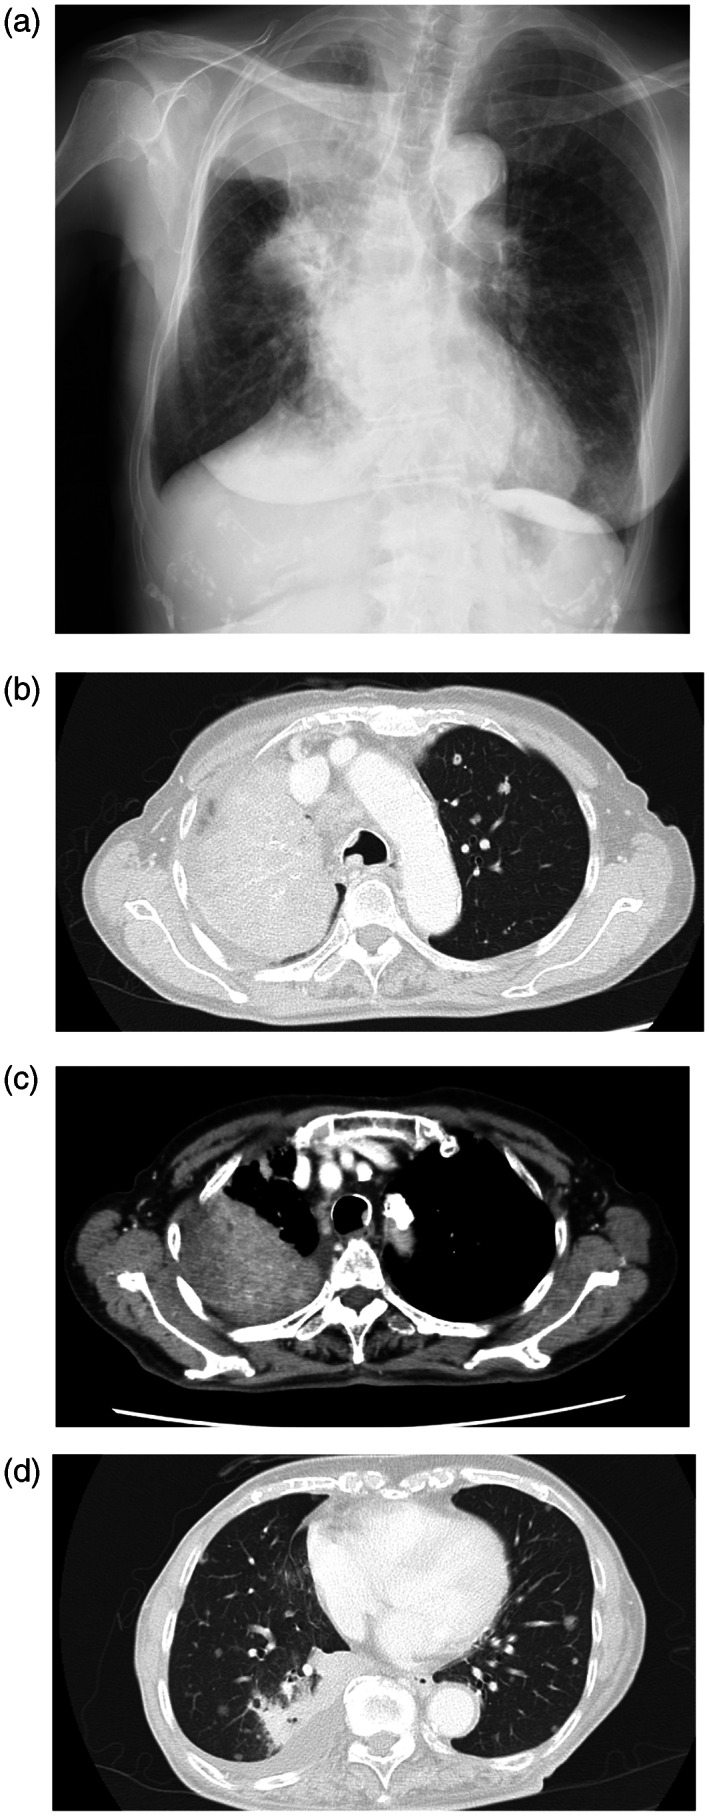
(a) Chest X‐ray and (b, c, d) contrast‐enhanced computed tomography (CT) at first admission. Mass shadows in the right upper, middle, and lower lobes, multiple nodular shadows in both lungs, and intramural nodules in the trachea and right bronchus. Right pleural effusion was noted.
